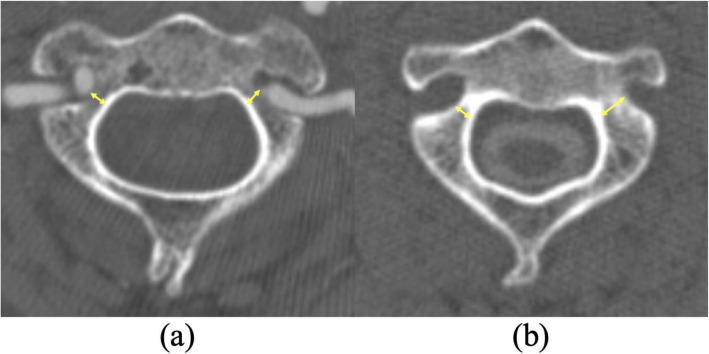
Pedicle diameter of the axis (C2) measured on axial computed tomography (CT). a Axial CT angiography. The pedicle diameter on both sides was <3.5 mm. b Axial CT myelography. The pedicle diameter on the left side was >3.5 mm, but <3.5 mm on the right side
